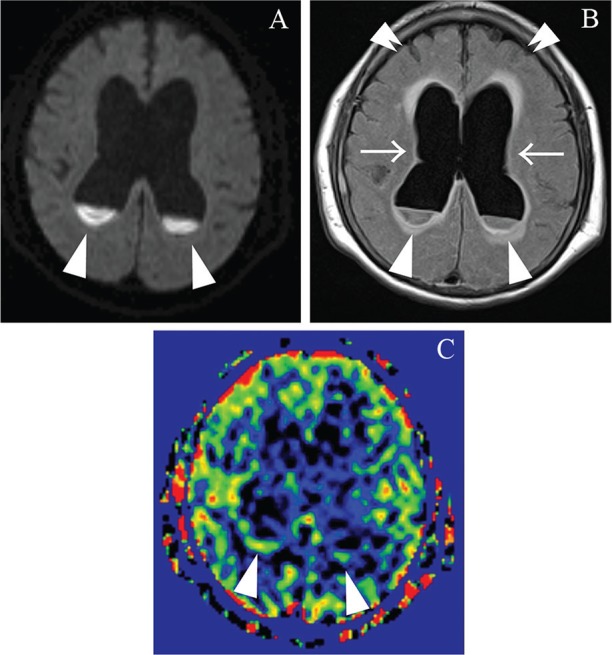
An 85-year-old woman with ventricular involvement due to unspecified bacterial infection. Diffusion weighted imaging and FLAIR show the purulent fluid collections as high intensity areas pooled in the posterior horns of the bilateral lateral ventricles (A, B: arrowheads). In addition, FLAIR demonstrates not only periventricular inflammation as periventricular high intensity areas in the ventricular walls, but also hydrocephalus as dilated ventricles (B: arrows) in conjunction with compacted sulci of the bilateral cerebral hemispheres (B: double arrowheads). Arterial spin-labeling magnetic resonance imaging shows bracket-like high perfusion along the posterior walls of the bilateral lateral ventricles (C: arrowheads). FLAIR, fluid-attenuated inversion recovery.
